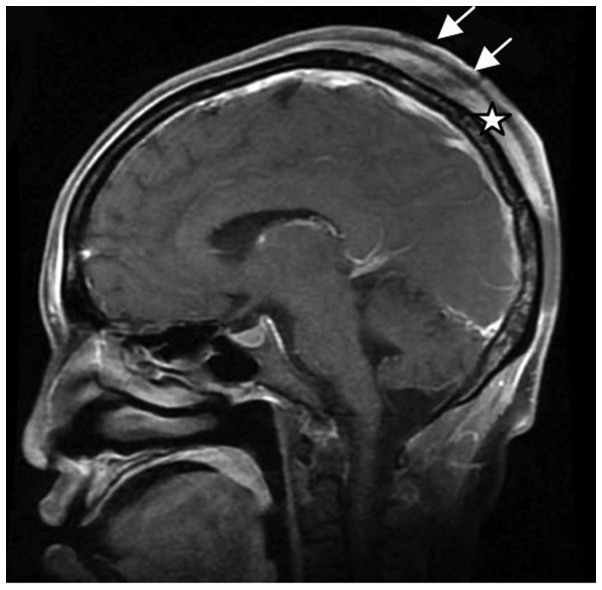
Midline sagittal T1-weighted image with fat-suppression following administration of contrast agents, captured on January 24, 2011. Image shows heterogeneous thickening of the galea aponeurotica, identified by the star and of the skin on the top of the brain with significant enhancement, as well as the dura mater of the parietal and occipital lobe. Defects are evident in the skin and are indicated by the white arrows. The diploë on the parietal and occipital bone is also shown to be heterogeneously enhanced.
